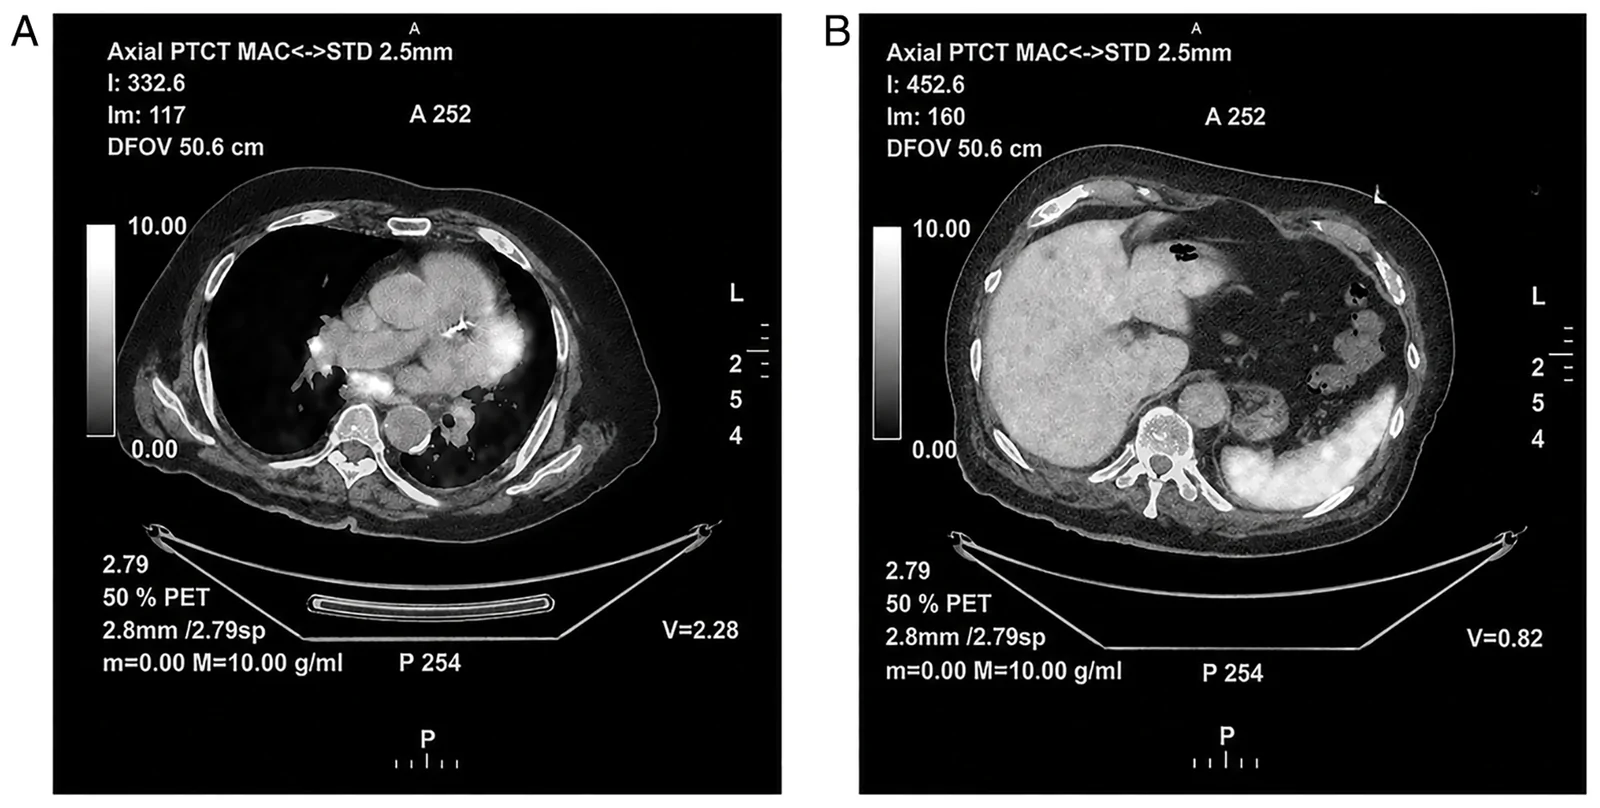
18F-FDG PET/CT images. (A) Axial chest image demonstrating hypermetabolic hilar/mediastinal lymph nodes associated with diffuse infiltrative-type pulmonary 18F-FDG uptake. (B) Axial upper abdominal image demonstrating diffusely increased splenic 18F-FDG uptake. 18F-FDG, axial fused fluorodeoxyglucose F 18.
